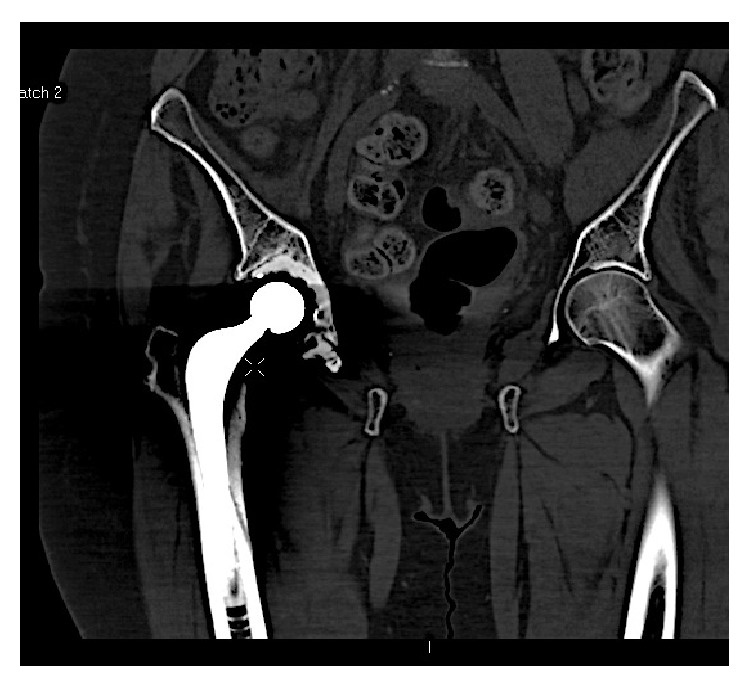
CT scan showing vertical split in right femoral shaft.
